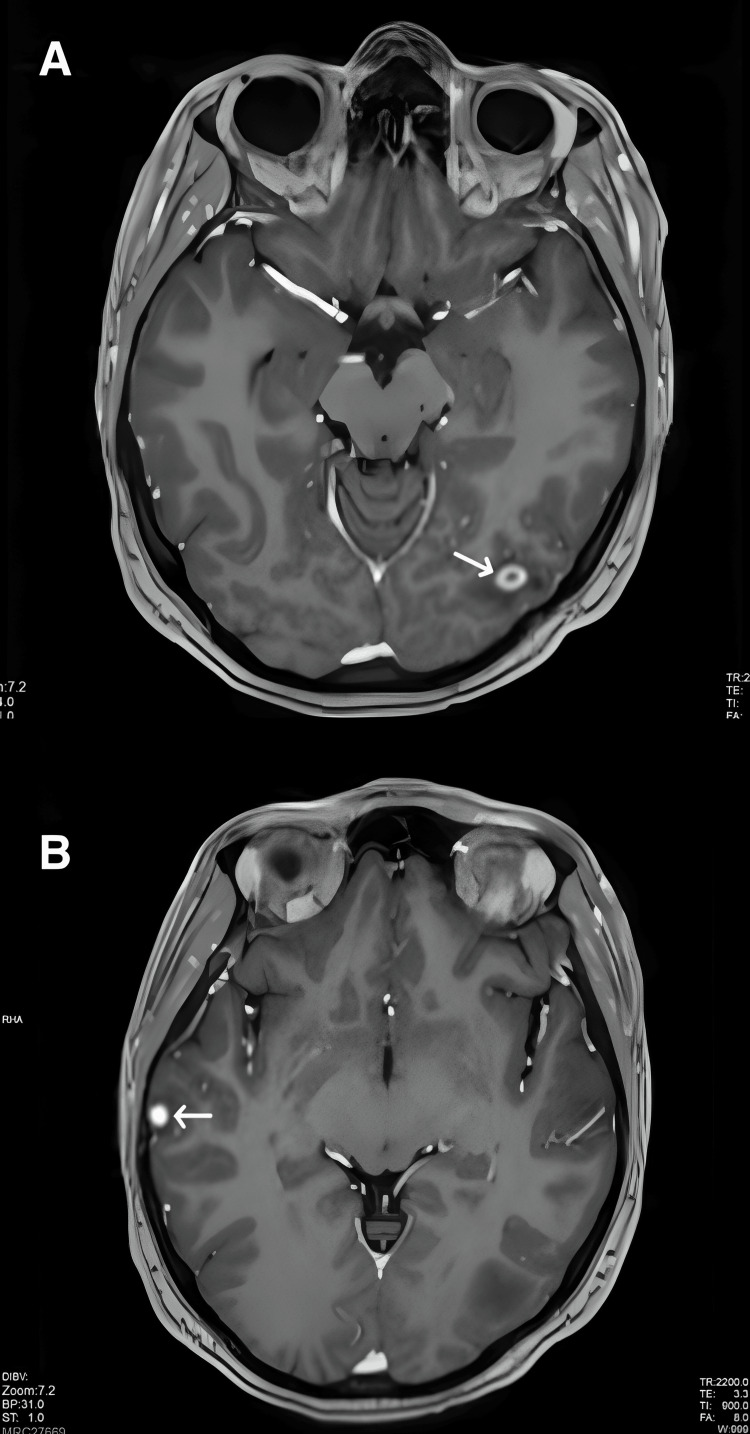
Contrast-enhanced MRI of the brain (A) Enhancing lesion in the left occipital lobe (white arrow). (B) Enhancing lesion in the right temporal lobe (white arrow).
